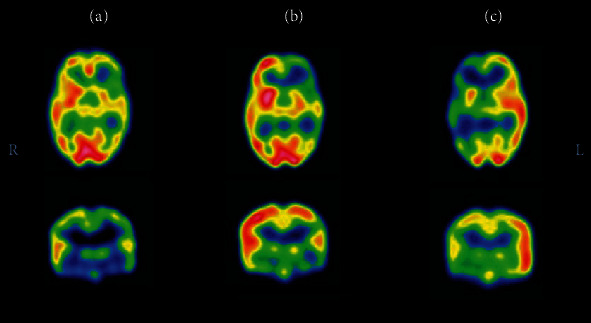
Ictal and interictal SPECT scans in a three-year-old boy with drug-resistant epilepsy. (a) Interictal SPECT showed prominent hypoperfusion over the left frontotemporal region. (b) First ictal SPECT attempt was performed in a seizure of 46-second duration, and radioisotope was injected at 40 seconds from the seizure onset. This was a late injection, and ictal SPECT showed similar findings as to the interictal SPECT. (c) Repeated ictal SPECT attempt was performed in a seizure of nine-second duration, and radioisotope was injected at two seconds from the seizure onset. This was an early injection, and ictal SPECT showed a relative increase in perfusion in the left frontotemporal region, compared with the right. This demonstrates the significance of early injection in accurately localizing the seizure onset zone, adapted from Kim et al. [38].
